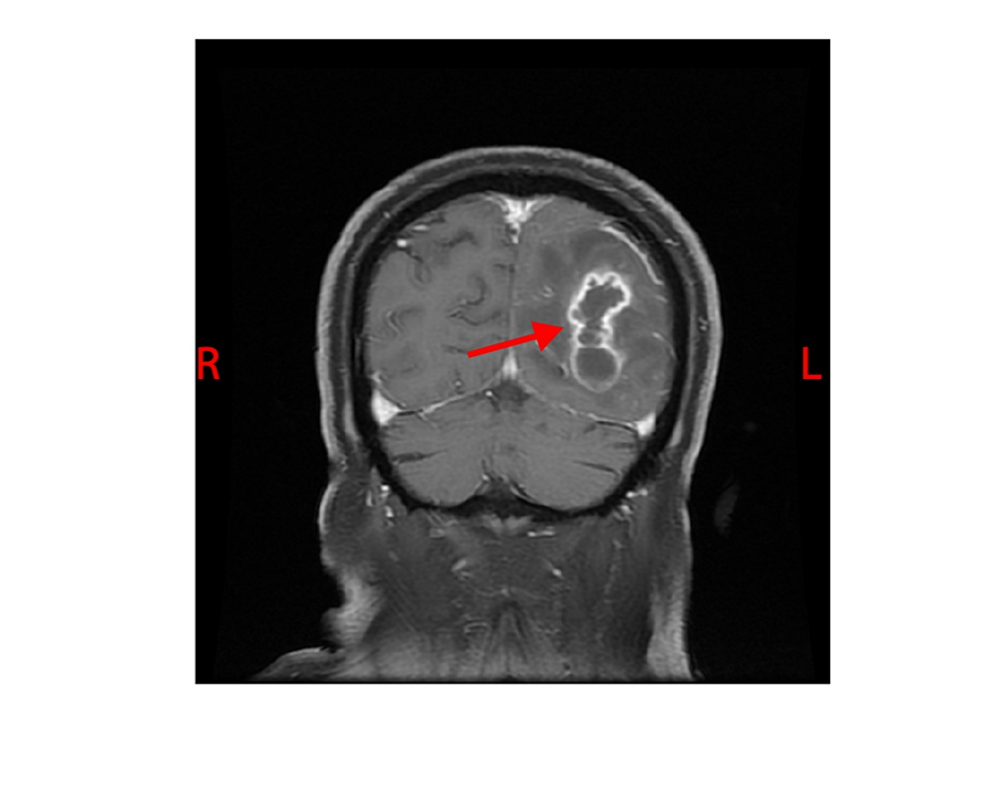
Coronal brain MRI with contrast demonstrated (red arrow) left parieto-occipital lesion.
